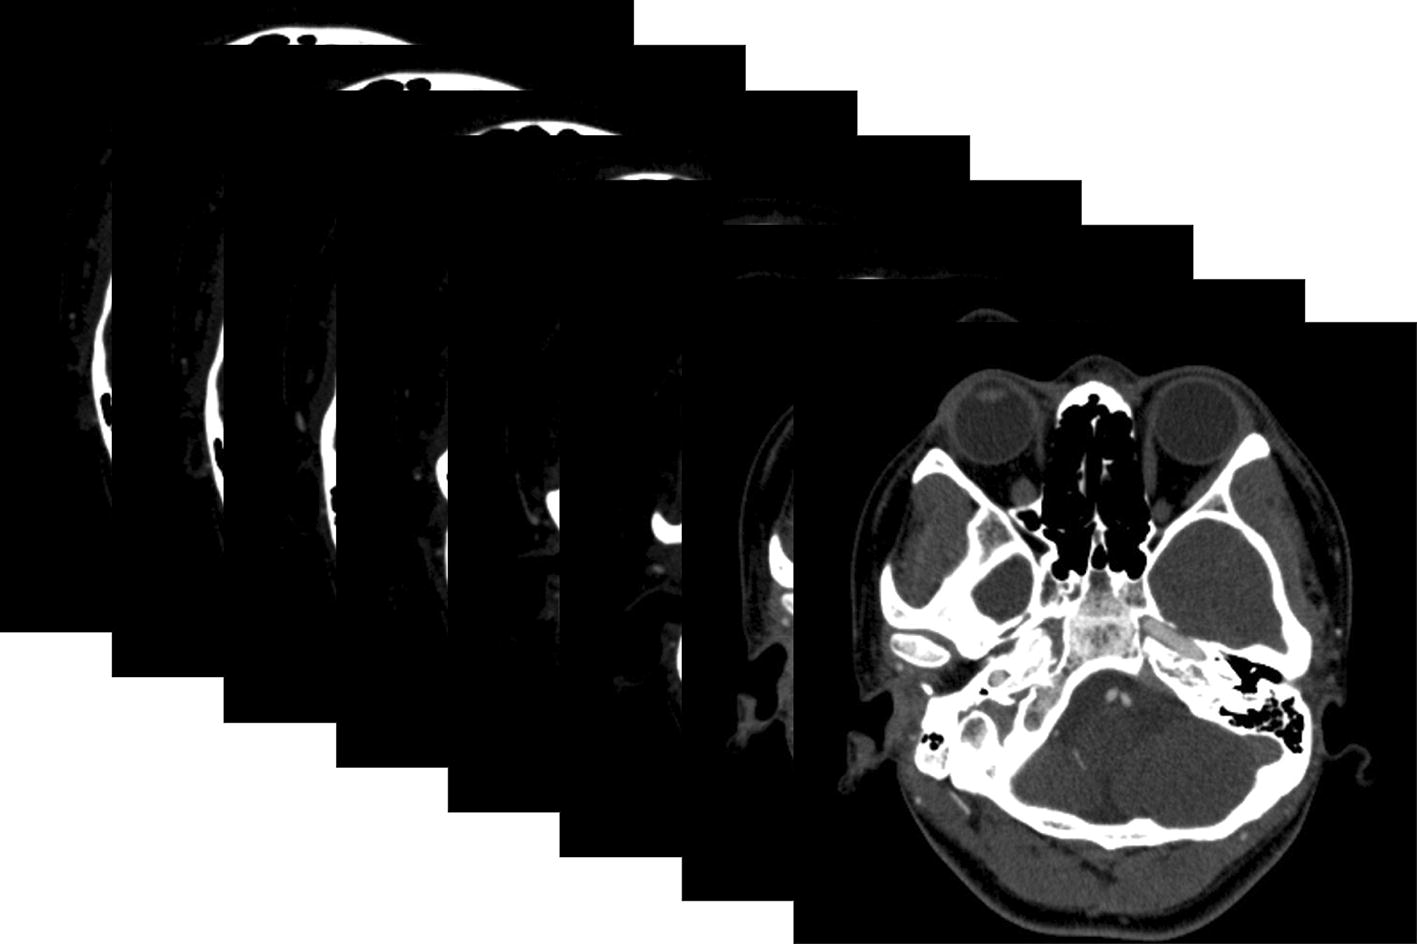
CTA image set of cerebral scanning
